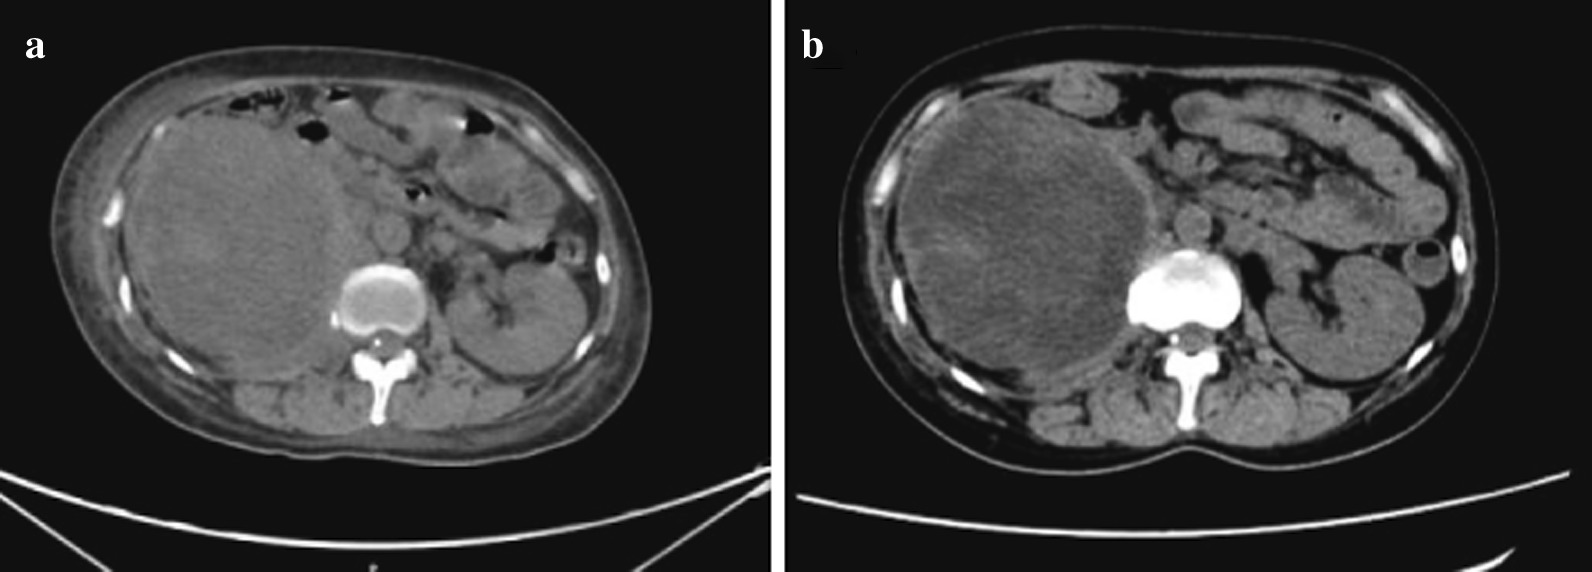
Abdominal CT scan performed before administration of the first dose of nivolumab (a), after administration of the third dose of nivolumab (b)
